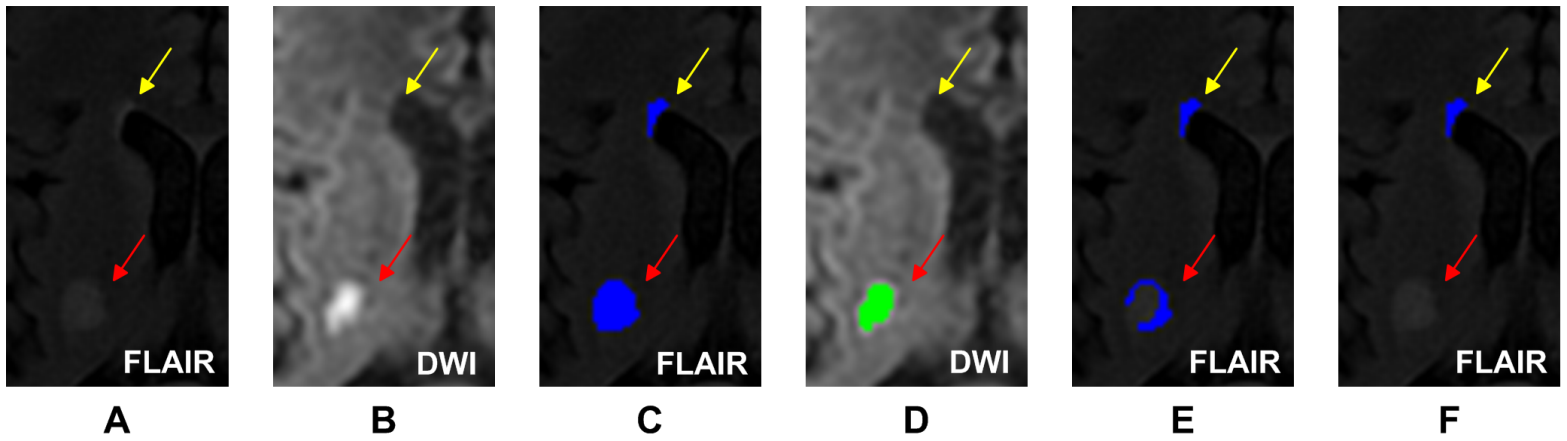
Elimination of a spurious WMHs due to postischemic peri-infarct edema. (A) The hyperintensities pointed to by the yellow and red arrows were WMHs and cerebral infarct, respectively, in FLAIR; (B) the infarct appeared hyperintensive in DWI, with a peripheral intensity reduction due to peri-infarct edema; (C) both regions were detected as WMHs in Step 10.2; (D) the green region pointed to by the red arrow was the infarct detected in Step 11.1; (E) the blue regions were the result of subtracting the result of Step 11.1 from the result of Step 10.2; the blue region pointed to by the red arrow was a spurious WMHs due to peri-infarct edema; (F) the result of Step 11.2; the spurious WMHs due to peri-infarct edema was eliminated successfully.
